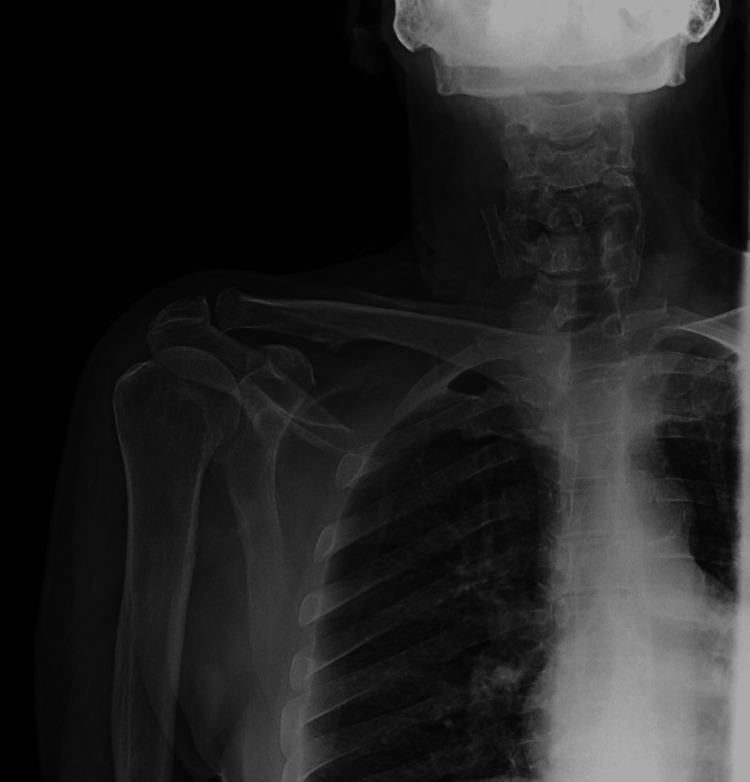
Shoulder X-ray Normal X-ray examination of the right shoulder, including normal glenohumeral articulation, acromioclavicular joint, acromion, and humeral head. The soft tissue structures are unremarkable. Normal visualized pulmonary apex.
